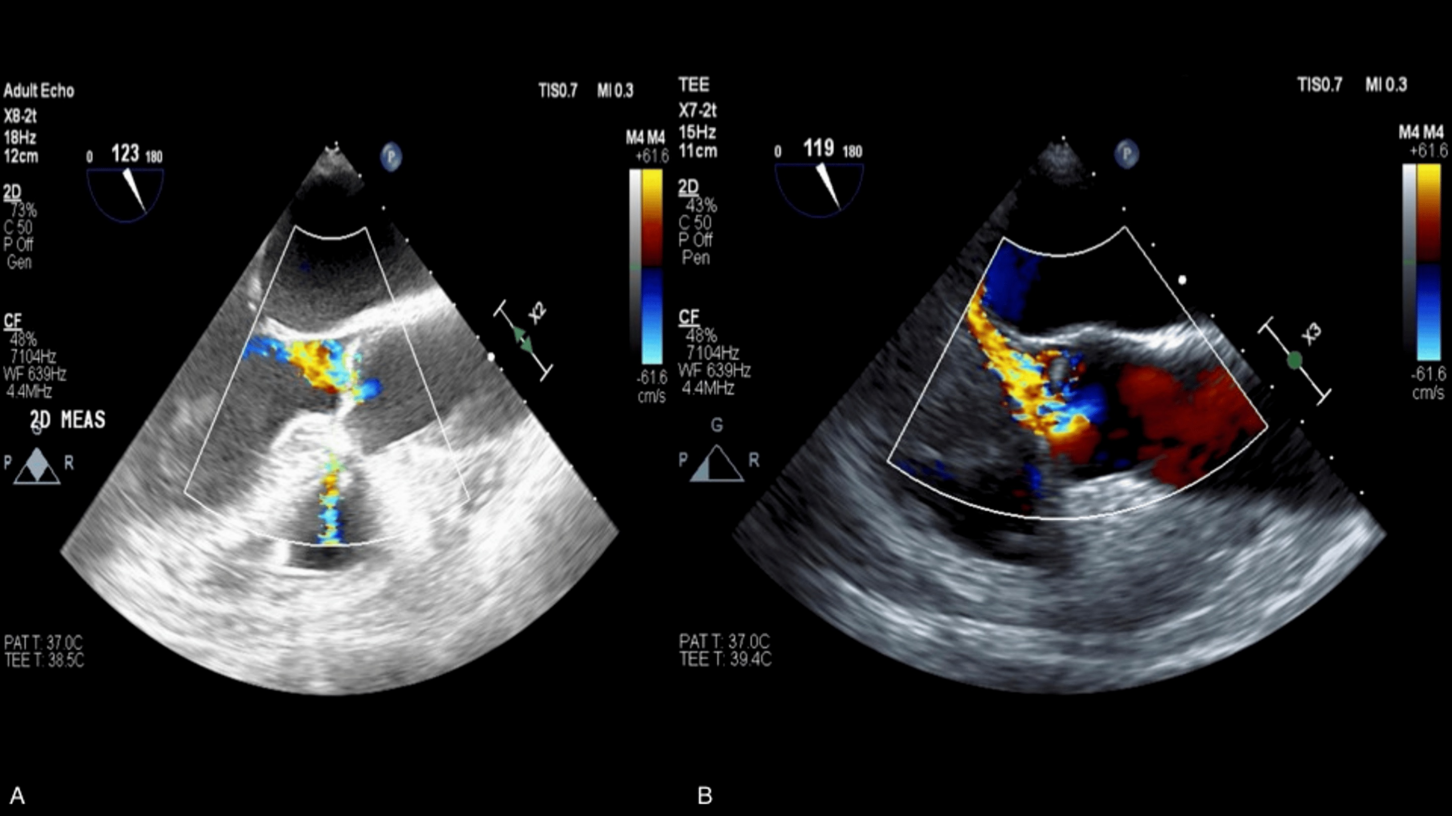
A transesophageal echocardiogram showing the mid-esophageal long-axis view at approximately 120° with color Doppler (A) Severe eccentric aortic valve regurgitation is seen at the noncoronary cusp, with leaflet bowing noted. (B) Severe eccentric aortic valve regurgitation is seen at the noncoronary cusp
